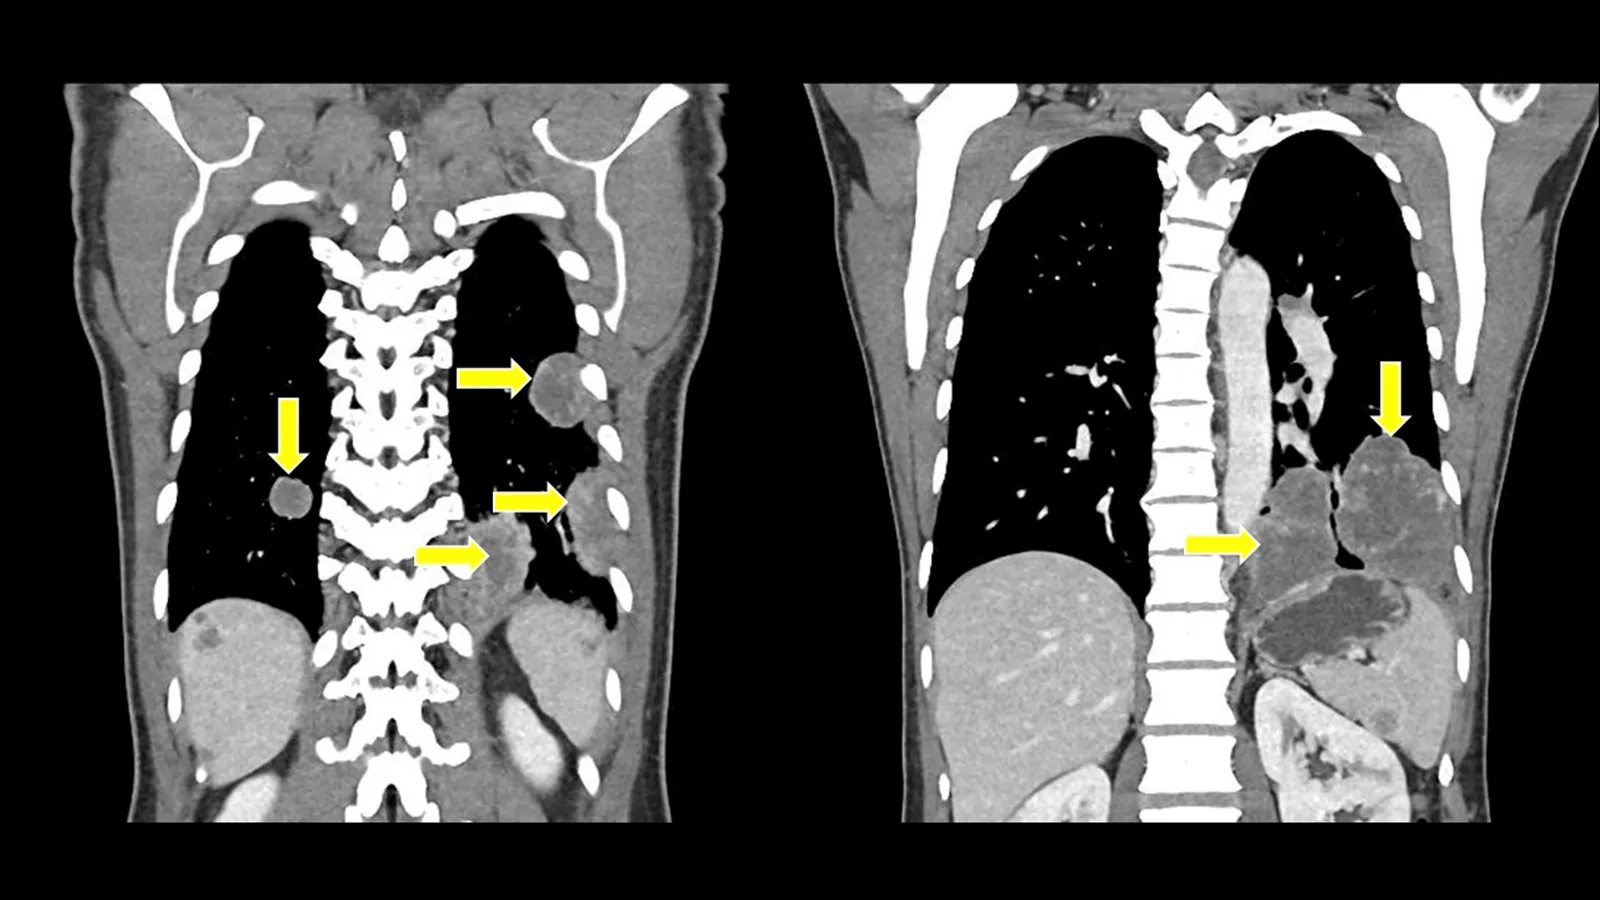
Contrast-enhanced CT of the chest Coronal contrast-enhanced CT images show multiple nodular masses, as seen on the chest X-ray CT: computed tomography
